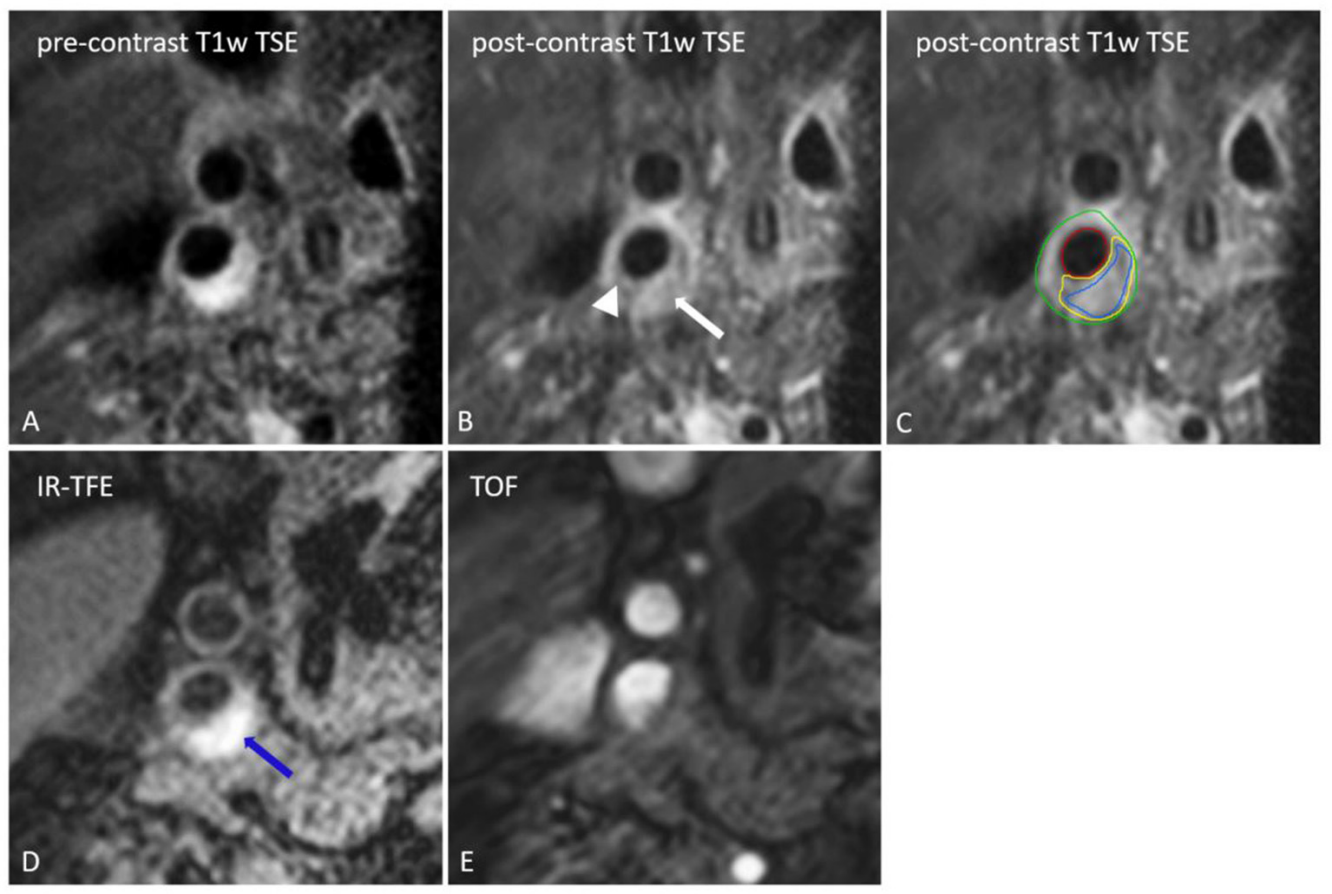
Transversal MR images of the right internal carotid artery. The black blood pre-contrast image (A) is used to draw the contours of the lumen and outer vessel wall. The lipid-rich necrotic core shows no contrast-enhancement on the post-contrast black-blood T1w quadruple inversion recovery (QIR) turbo spin echo (TSE) image (B) and includes the entire area of hemorrhage (IPH) [IPH: blue, lipid-rich necrotic core: yellow, lumen: red, outer vessel wall: green on (C)]. IPH [blue arrow on (D)] appears as a bright signal on the inversion recovery turbo field echo images (IR-TFE; D). A thin or ruptured fibrous cap (TRFC) can be identified by the interruption of juxtaluminal signal enhancement on the post-contrast T1w image (arrow head). With a contra-indication for contrast injection the T2w image or time of flight (TOF) image (E) can be used for TRFC assessment.
